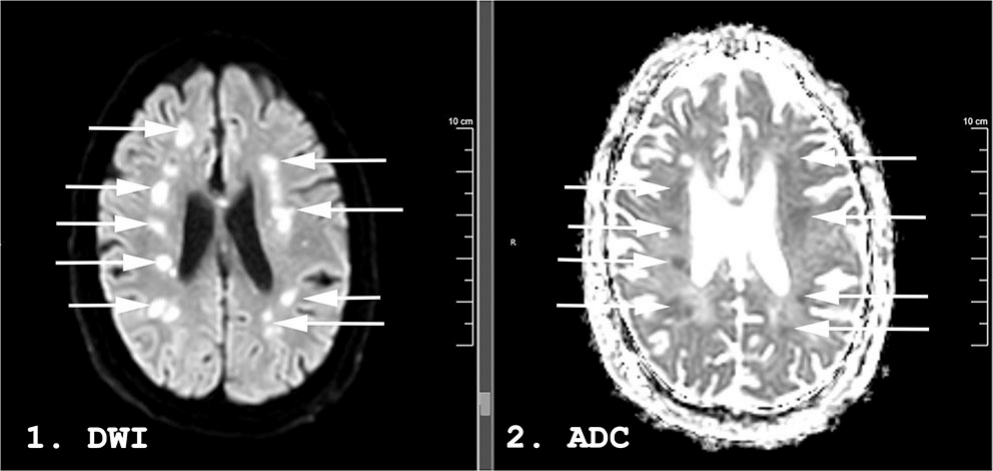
Magnetic resonance imaging (MRI) performed 31 days following admission on a 59-years-old man with COVID-19. Diffusion Weighted Imaging (DWI) in panel 1 and apparent diffusion coefficient (ADC) in panel 2. Hyperintense lesions are seen in the bilateral middle/anterior cerebral artery (MCA-ACA) borderzones on DWI (arrows). Some demonstrate a hypointense ADC correlate (arrows) while others are isointense on ADC, suggesting an acute to subacute timeframe.
